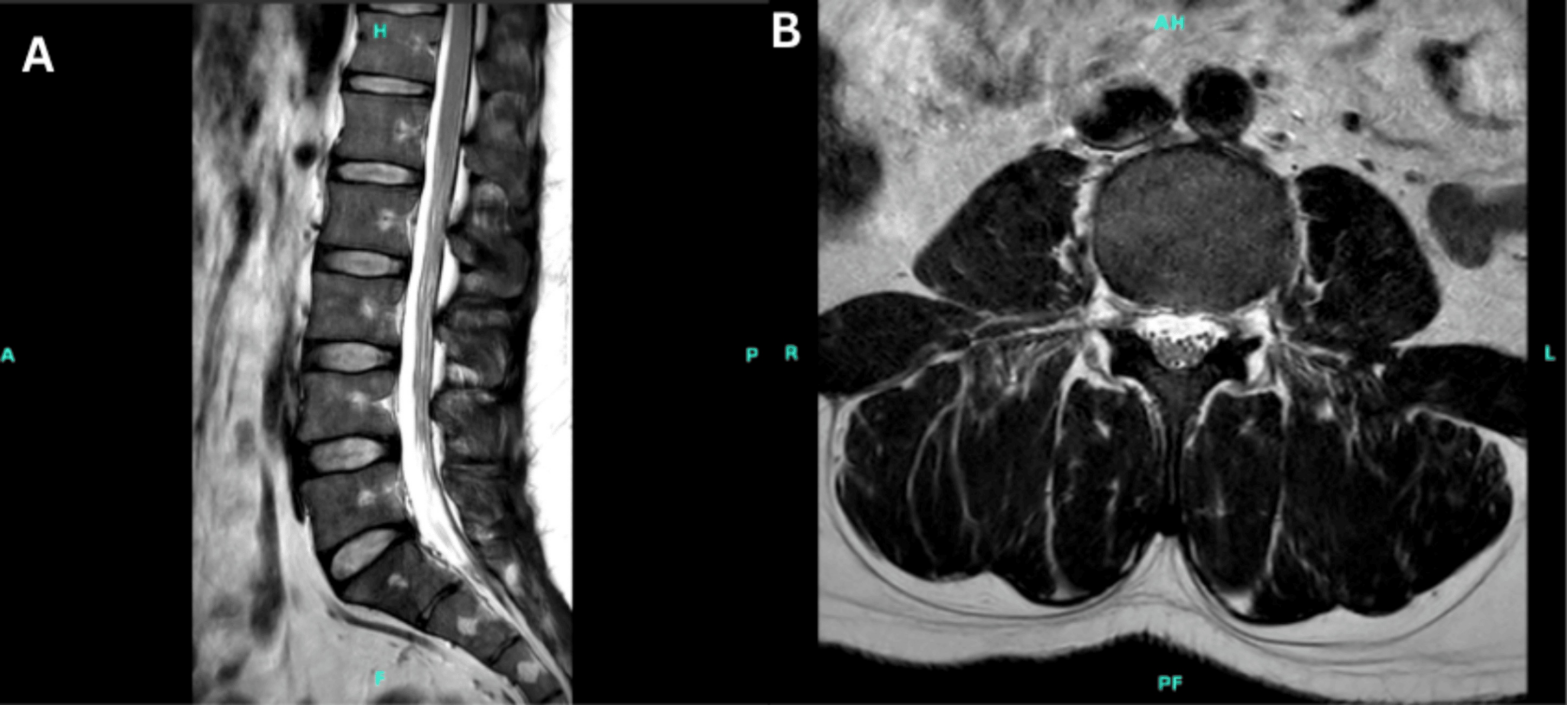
MRI of the Lumbar Spine WO (A) The axial T2-weighted image demonstrates a normal spinal canal, no disc herniation, and patent neural foramina. (B) Sagittal T2-weighted image shows normal alignment, preserved disc height, and no evidence of central canal stenosis or nerve root compression. These findings effectively exclude structural causes of radiculopathy.
